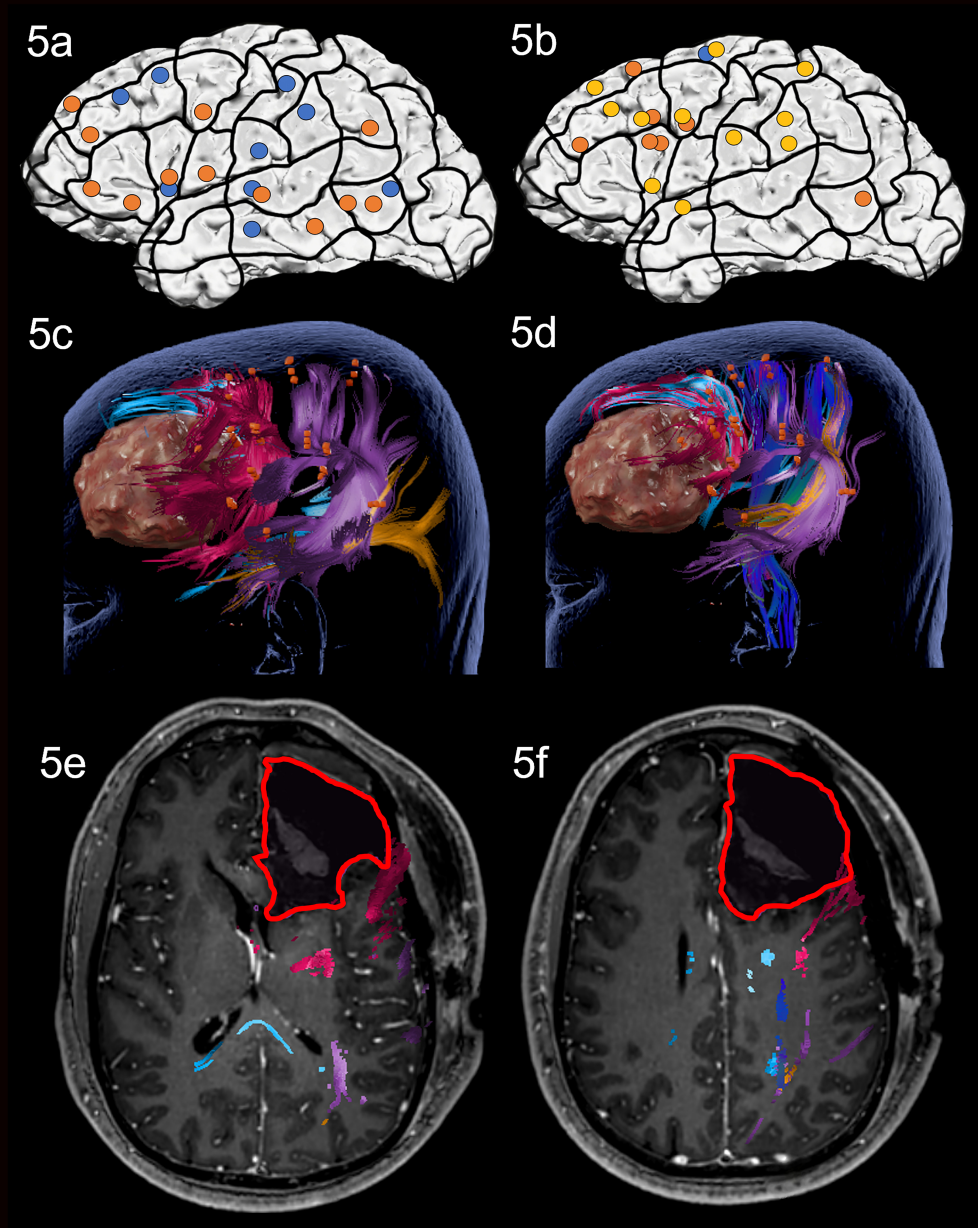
Case 5. Left panels show ON results, right panels AN results. Panels (A, B) nTMS stimulation sites that induced errors; no response and hesitations in orange, semantic errors in yellow and performance errors in blue. Panels (C, D) Fiber tracking results including the lesion, nTMS cortical endpoints in orange and color-coded tracts with CNT in direction-coded, here dark blue, AF in purple, ILF in yellow, Frontal Aslant Tract in pink, and CF in light blue. Panels (E, F) Overlap of tracking and postoperative T1-weighted imaging after elastic fusion with the resection cavity marked in red.
